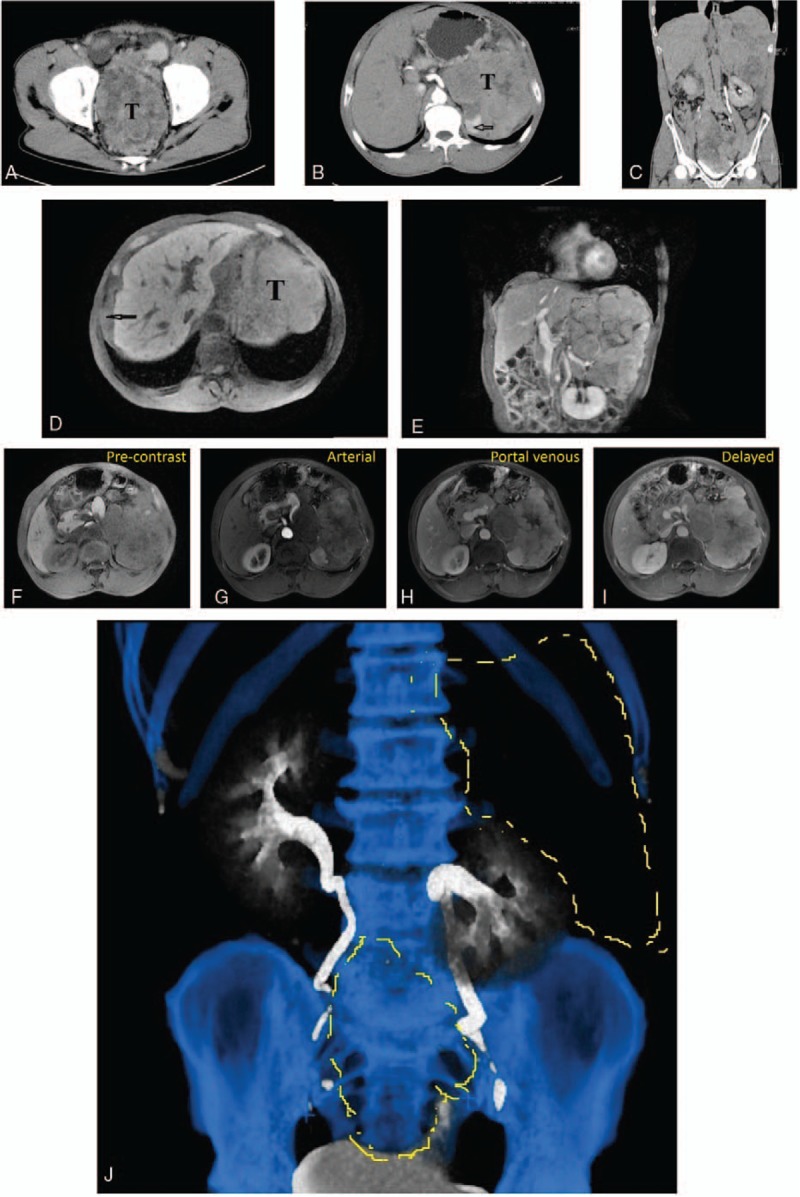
(A–C) Contrast-enhanced computed tomography of the entire abdomen found multiple masses located in left upper quadrant, hepatogastric gap, and pelvic cavity with irregular margin, heterogeneous density, and significant enhancement, involved to the spleen (arrow in B) and bladder. (D, E) Magnetic resonance imaging (MRI) of upper abdomen revealed a huge tumor (T) compressed the left kidney in the left upper quadrant without any communication with left liver lobe, another sharp edged soft tissue mass (arrow) located between the right liver and diaphragm. (F–I) MRI results showed masses presented with heterogeneous hyperenhancement in arterial phase, and more obvious hyperenhancement can be seen in portal venous and delayed phase, no washout of the contrast agent was detected. (J) CT urography revealed pressure on the bottom of the left ureter and left kidney.
